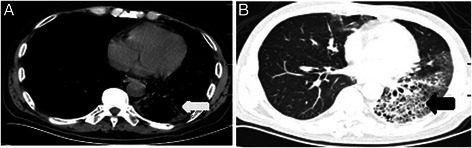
CT scan results. Computed tomography scan of the chest taken post-radiotherapy on December 21, 2012 (large arrow in a and b) showing: central type cancer of the left lung, radiation pulmonary fibrosis
